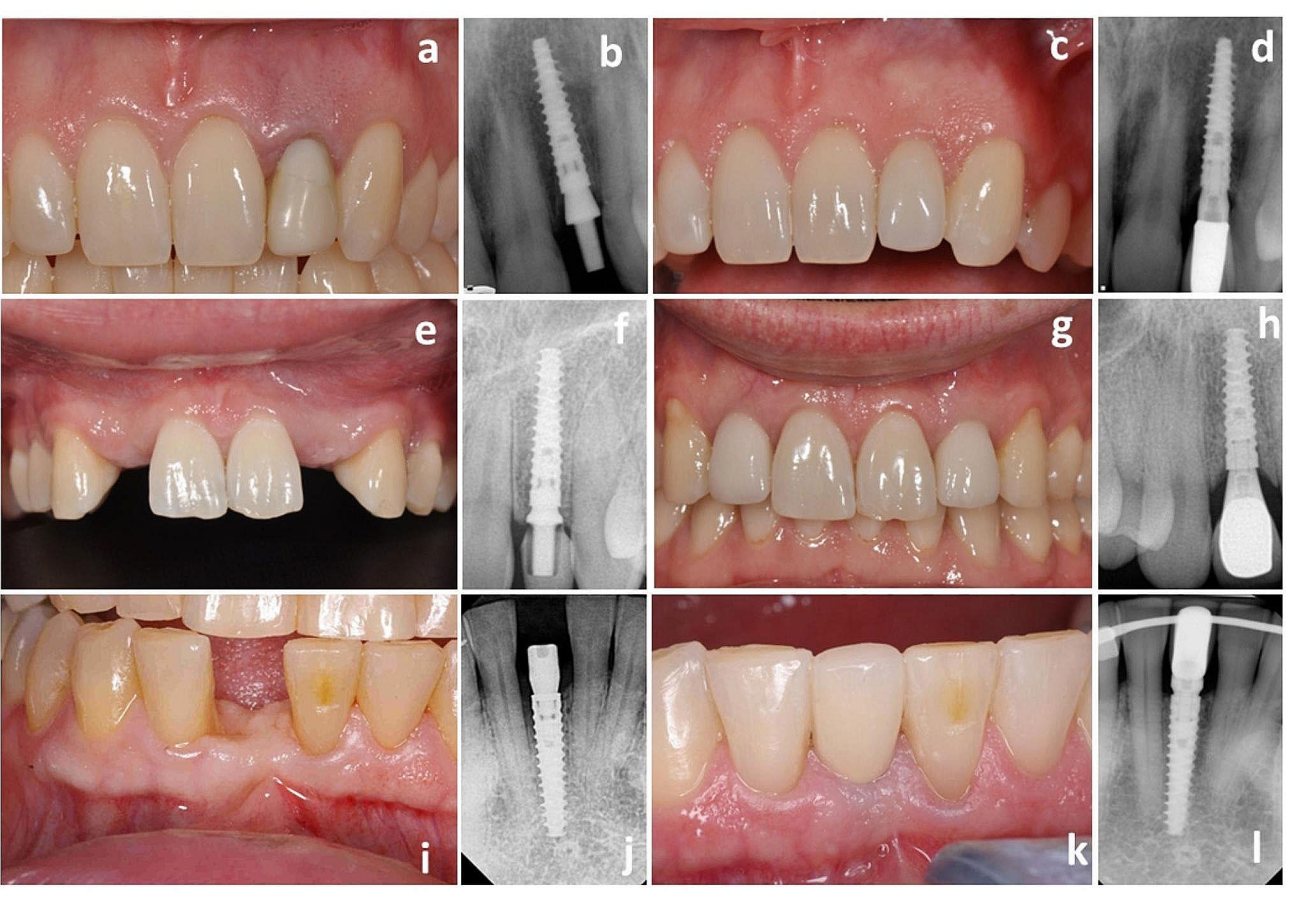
Clinical picture and radiographs of patients before treatment, at implant insertion, and 5 years later. Column on the left, images from pretreatment (a, e, i), center left, radiographs taken at the insertion of narrow (3-mm-diameter) implant (b, f, j), center right, photos at the 5-year follow-up visit after implant placement (c, g, k), and on the right, corresponding radiographs (d, h, l). Top row, a 38-year-old male (a, b, c, d) with a hopeless dentition at the lateral incisor in the maxilla (FDI position 22) received a 15-mm-long implant in the fresh socket. Middle row, a 27-year-old male (e, f, g, h) missing lateral incisors in the maxilla (FDI positions 12 and 22) received 13/15-mm mm long implants. Bottom, a 61-year-old female with a missing central incisor in a healed site of the mandible (i, j, k, l) received a 15-mm long implant
